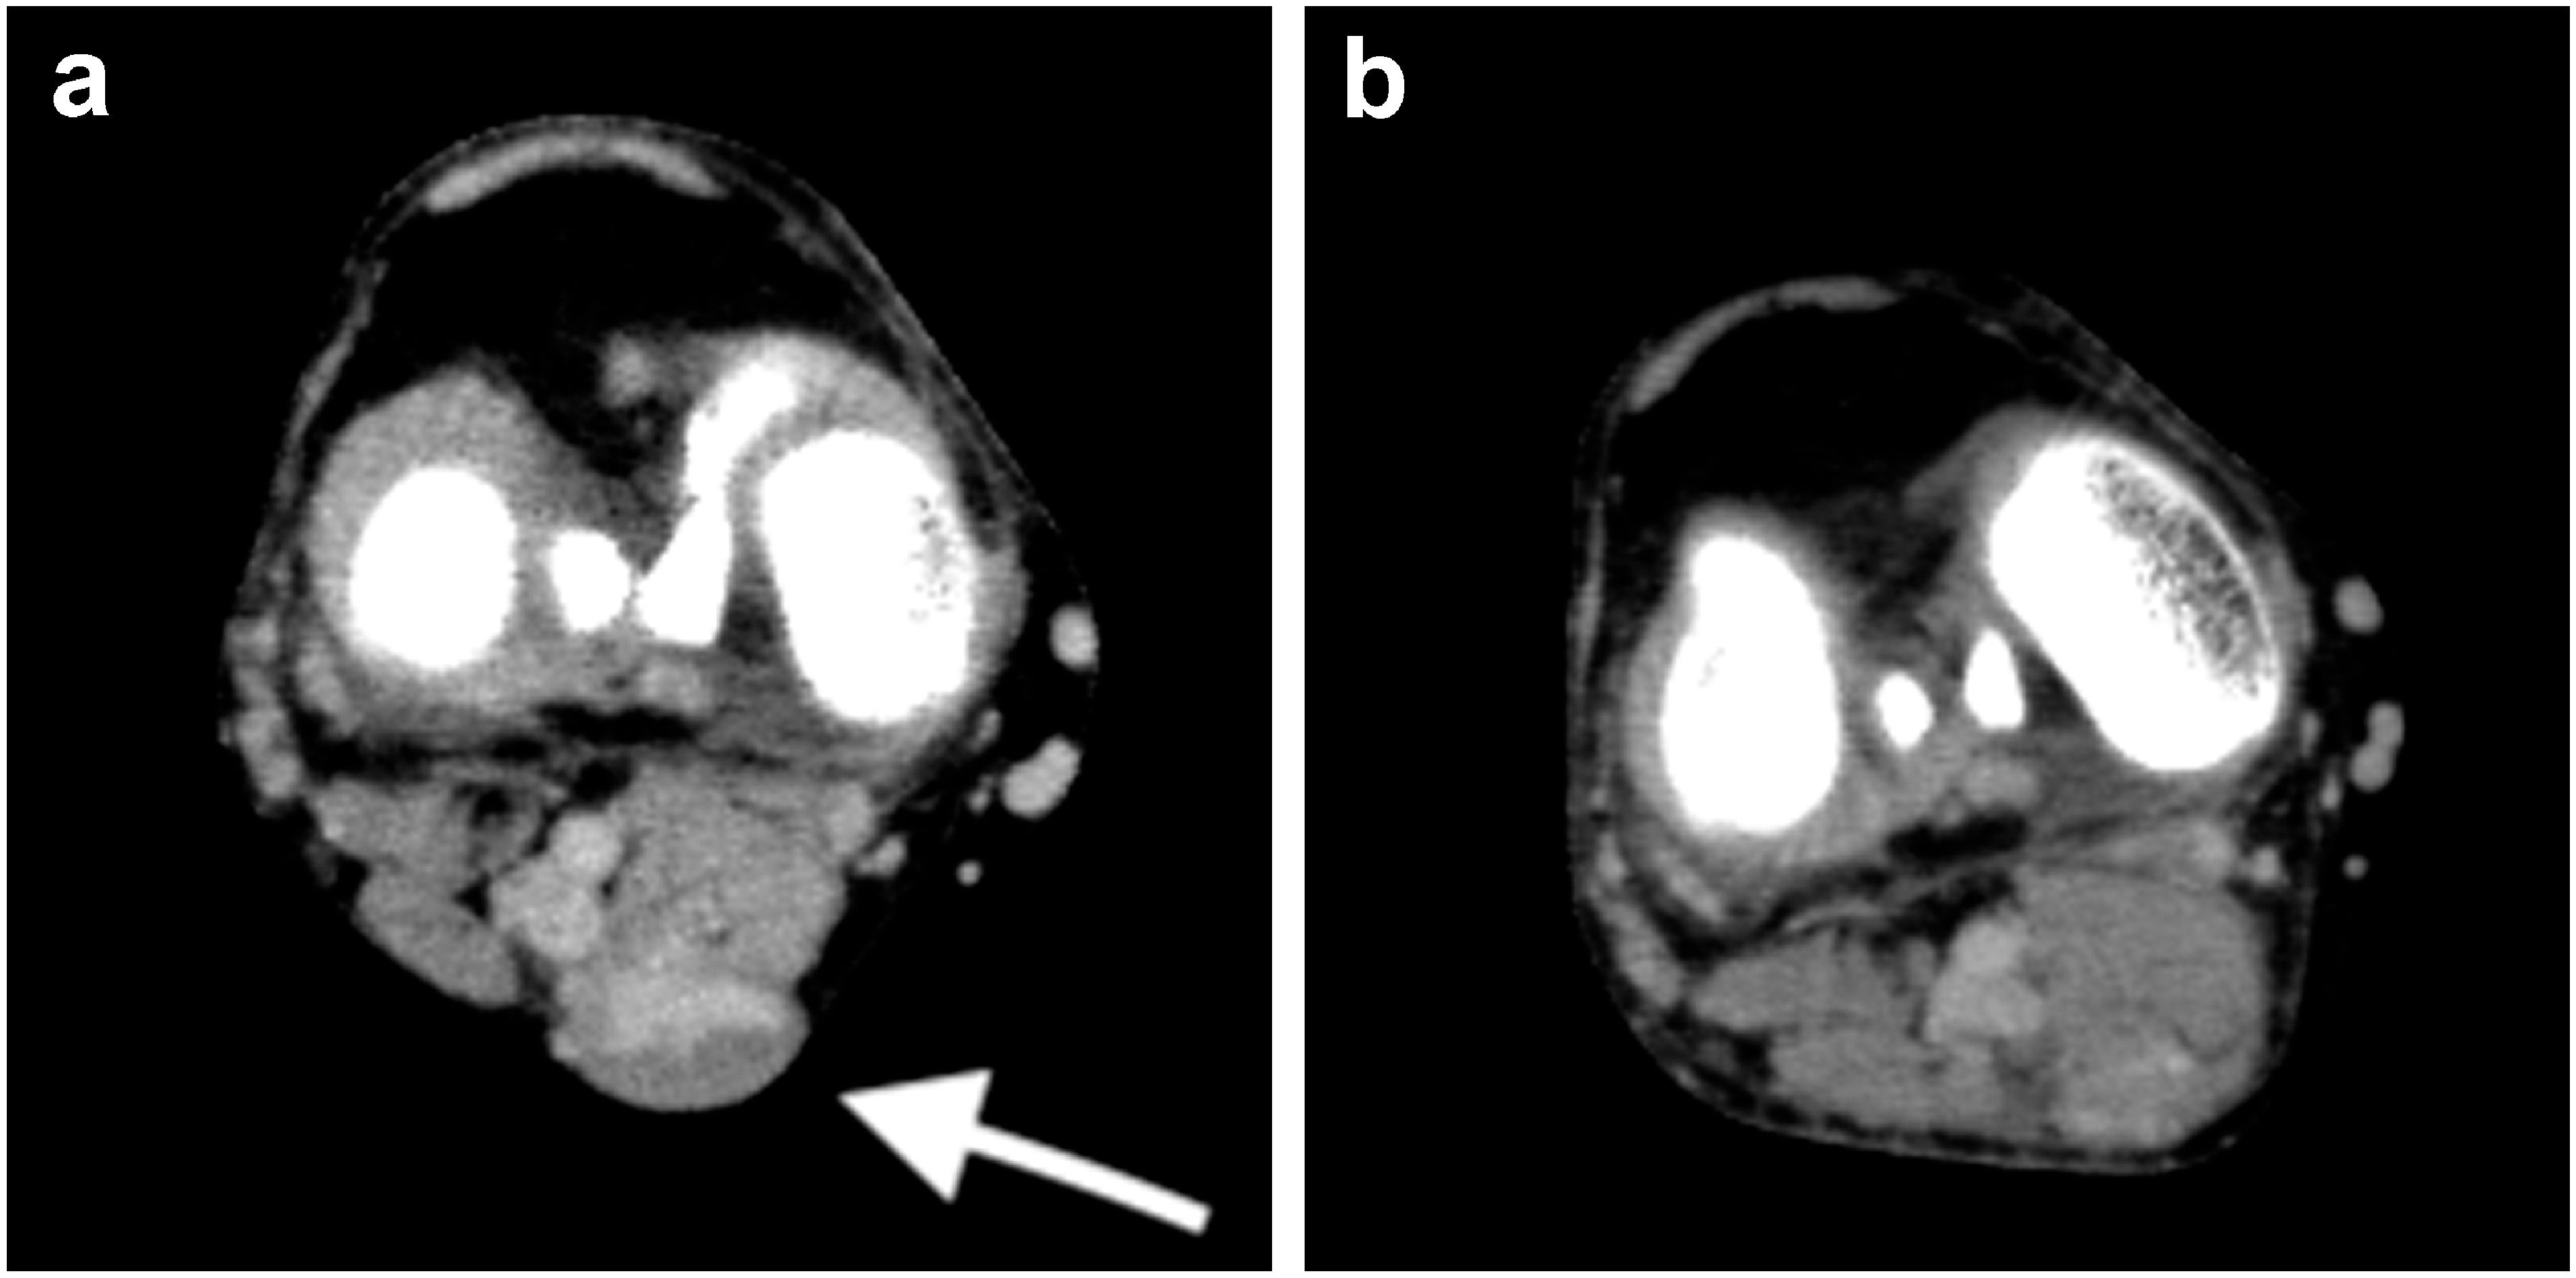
Fig. 2 (a) Preoperative contrast-enhanced computed tomography showed a saccular sural venous aneurysm on the right side with thrombus (white arrow). (b) Postoperative contrast-enhanced computed tomography showed no recurrent sural venous aneurysm in the right side of the popliteal fossa.
